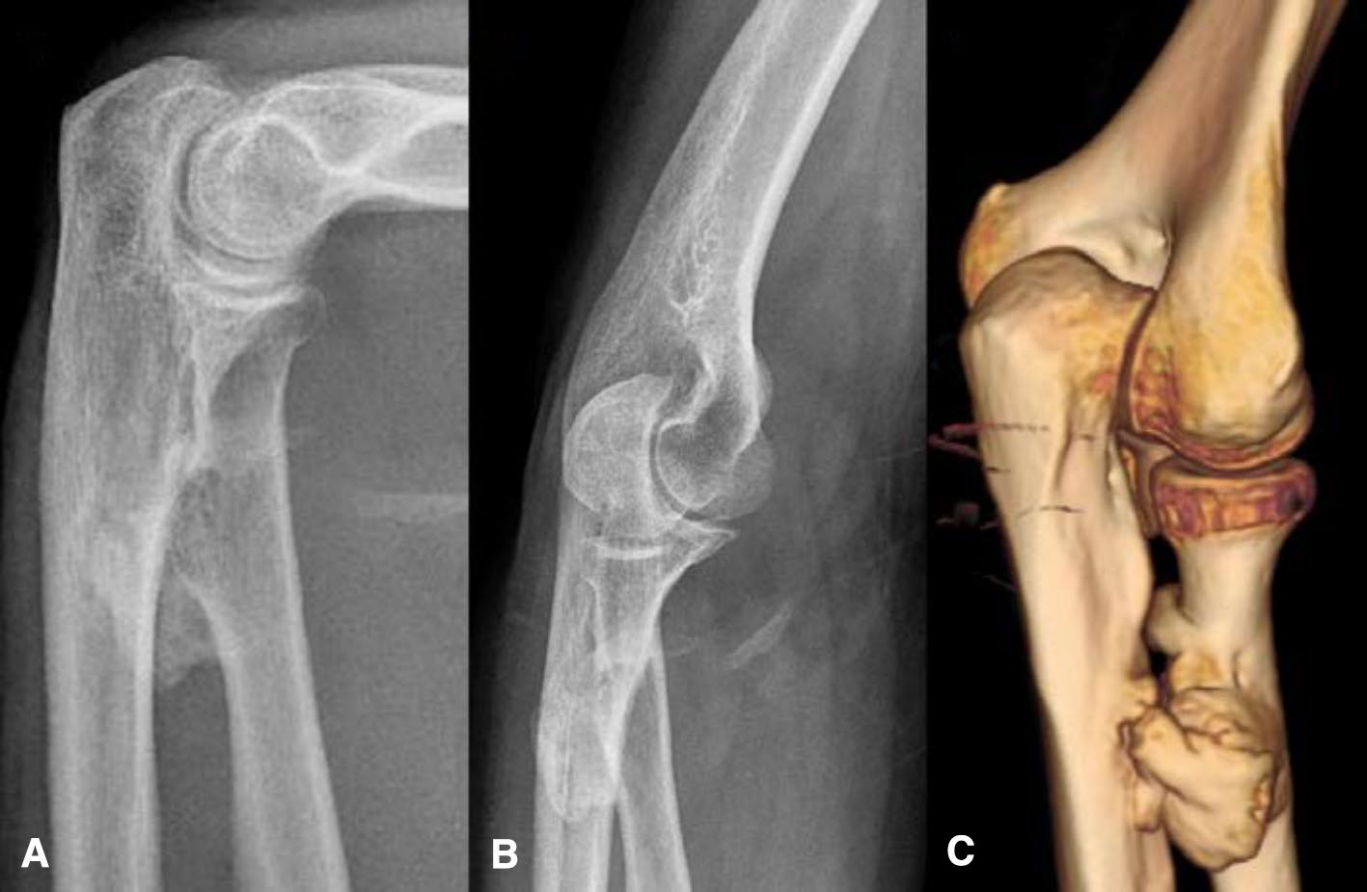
Proximal radioulnar synostosis: a–b antero-posterior and lateral X-ray views; c 3D TC reconstruction
